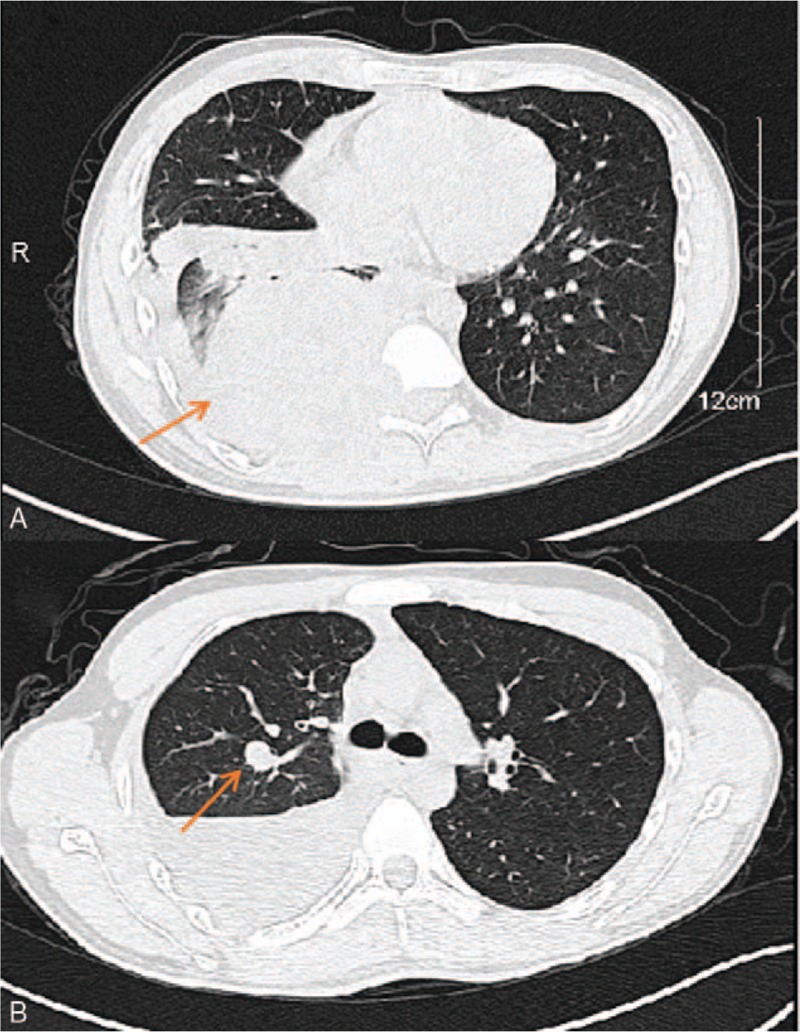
Axial CT study showing the soft tissue mass that involves the adjacent vertebrae (A) and metastasis in the posterior segment of the upper lobe of the right lung (B). CT = computed tomography.
